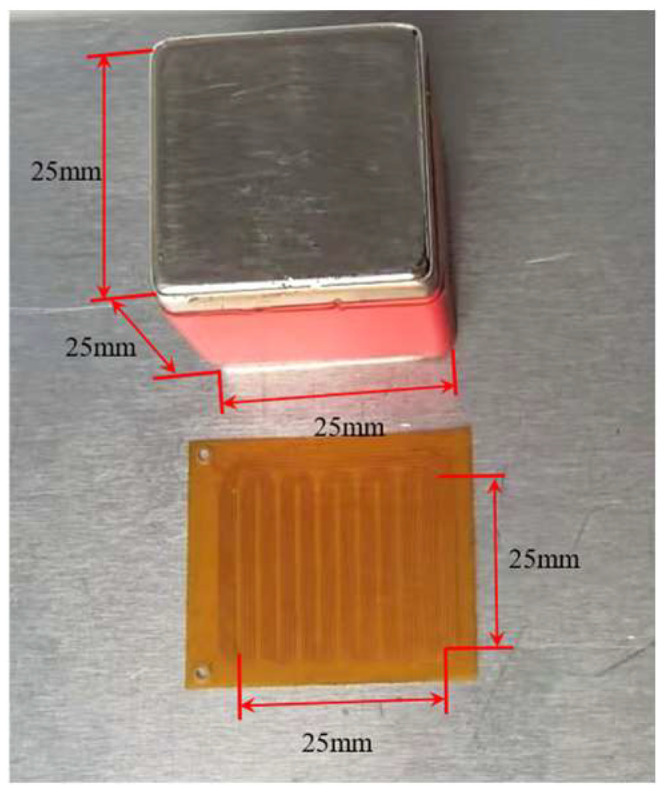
New electromagnetic ultrasonic surface wave transducer.
